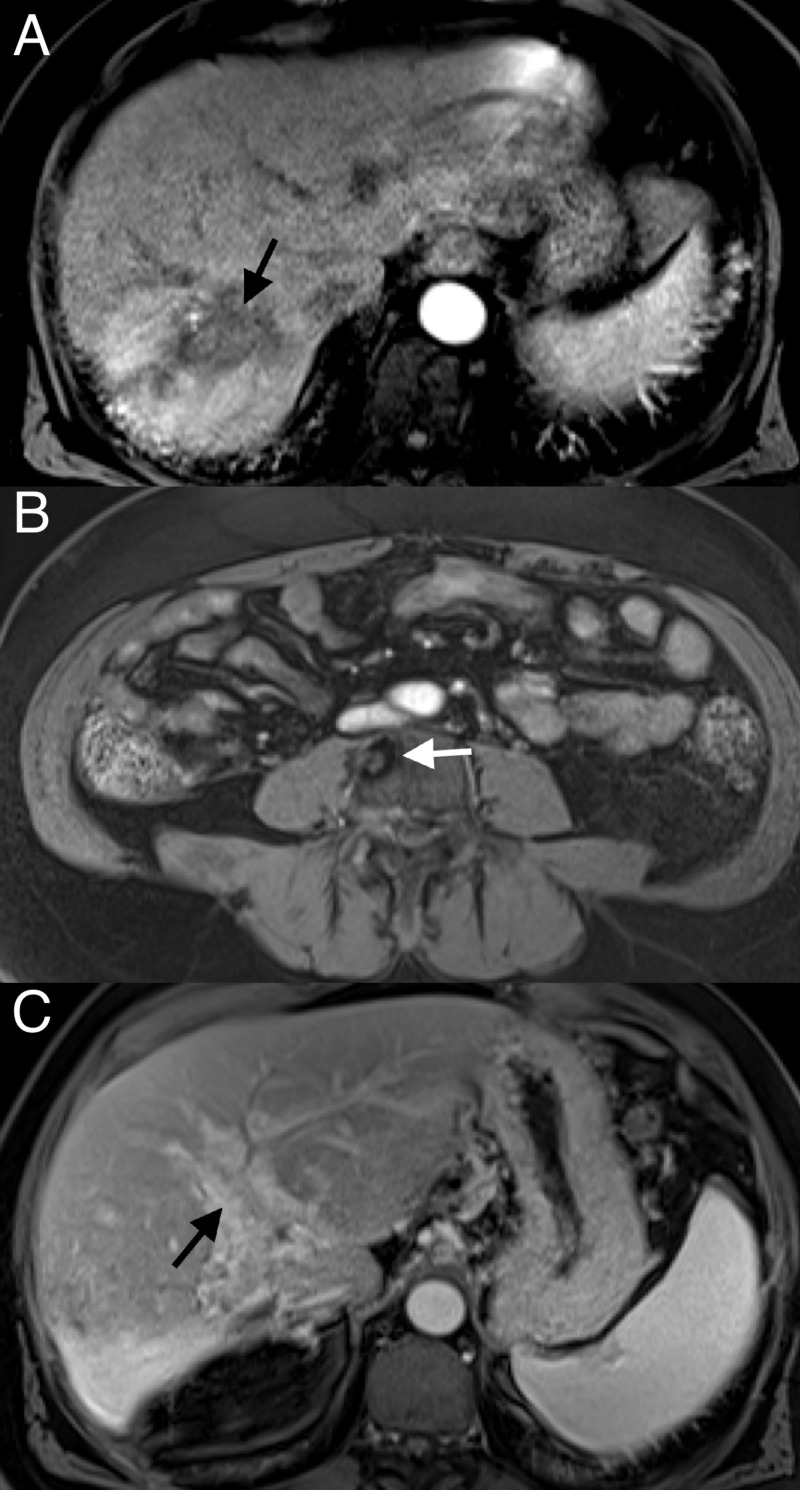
June 2018 MRI with IV contrast arterial phase 20 second (T1) vibe sequence (A) Further decrease in tumor size; (B) decreased enhancement of L4 lesion; (C) venous phase 70-second (T1) vibe sequence showing cavernous transformation of the portal vein and no clear evidence of residual tumor thrombus (chronic occlusion) MRI: magnetic resonance imaging, IV: intravenous
